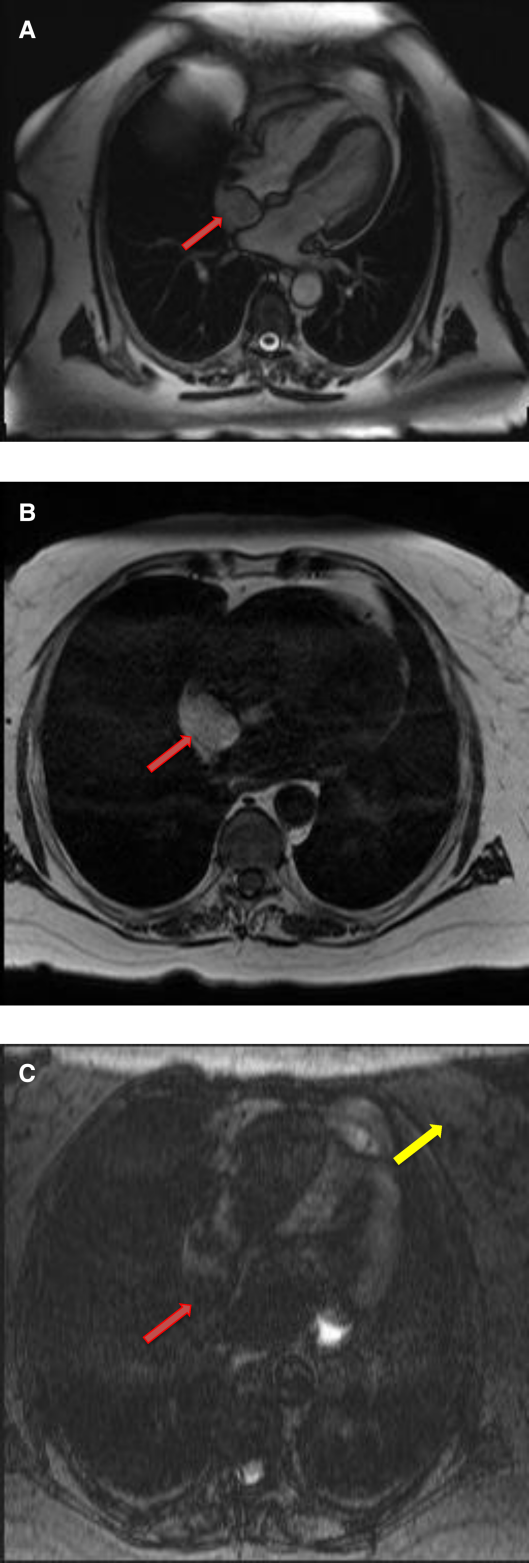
A 68-year-old female with intra-atrial septal lipoma. (A) (On line video 3), CMR cine images showing a sharply marginated mass arising from the intraatrial septum (red arrow). (B) This mass has same signal intensity (high) as surrounding fat on axial T1-weighted, dark blood, double inversion recovery fast spin echo images (red arrow). (C) Fat suppression sequence representing suppression of surrounding fat (white arrow) as well as the mass in the intra-atrial septum (red arrow).
